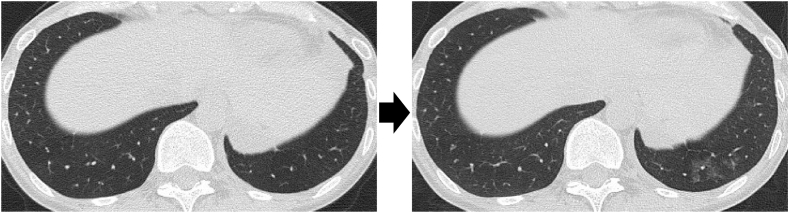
Changes in the Chest CT after the humidifier provocation test.
